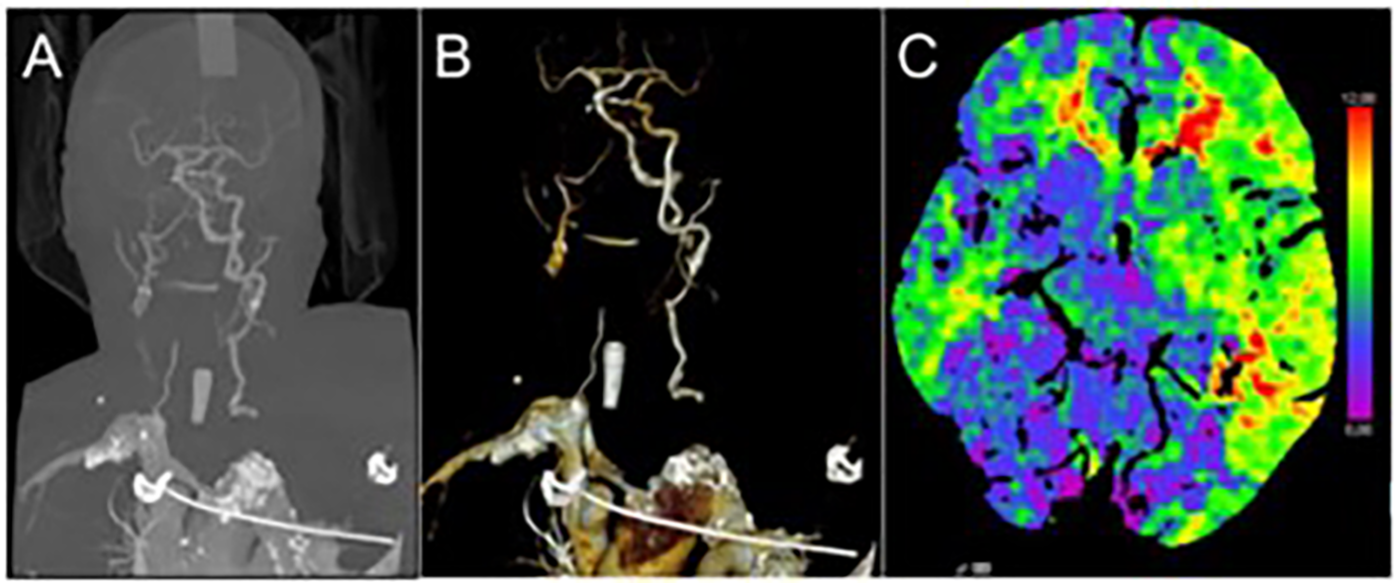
Preoperative contrast-enhanced computed tomography of the supra-aortic rteries showing long-segment antegrade obstruction of both carotid arteries and the right vertebral artery (A,B) and cerebral perfusion deficit predominately over the right cerebral hemisphere (C).
